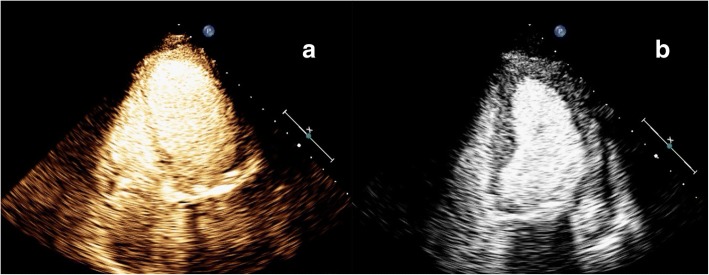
Myocardial contrast echocardiography (MCE) performed on the second day after surgery (a) and two weeks later (b). Both myocardial contrast echocardiography (MCE) examinations showed homogeneous and equal enhancement intensity in the basal, middle and apical segments in the four-chamber view
